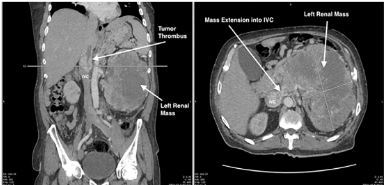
Computed tomography abdomen and pelvis shown in the coronal (left) and axial (right) view demonstrating a large heterogeneous structure measuring approximately 20 × 14 × 19 centimeters and extending into the inferior vena cava.
